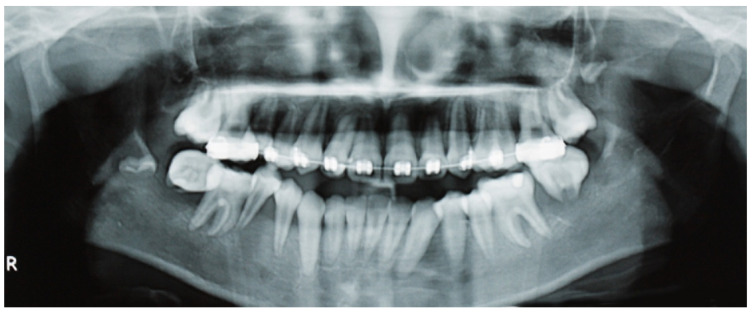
Panoramic radiography shows ill-defined radiolucencies in the posterior mandible bilaterally, affecting the adjacent permanent teeth that resemble a ‘floating teeth’ appearance. In this particular focal trough, the osteolysis appears to be more diffuse in the right mandible, extending into the premolar region and also causing the displacement of the sperm of the third molar.
